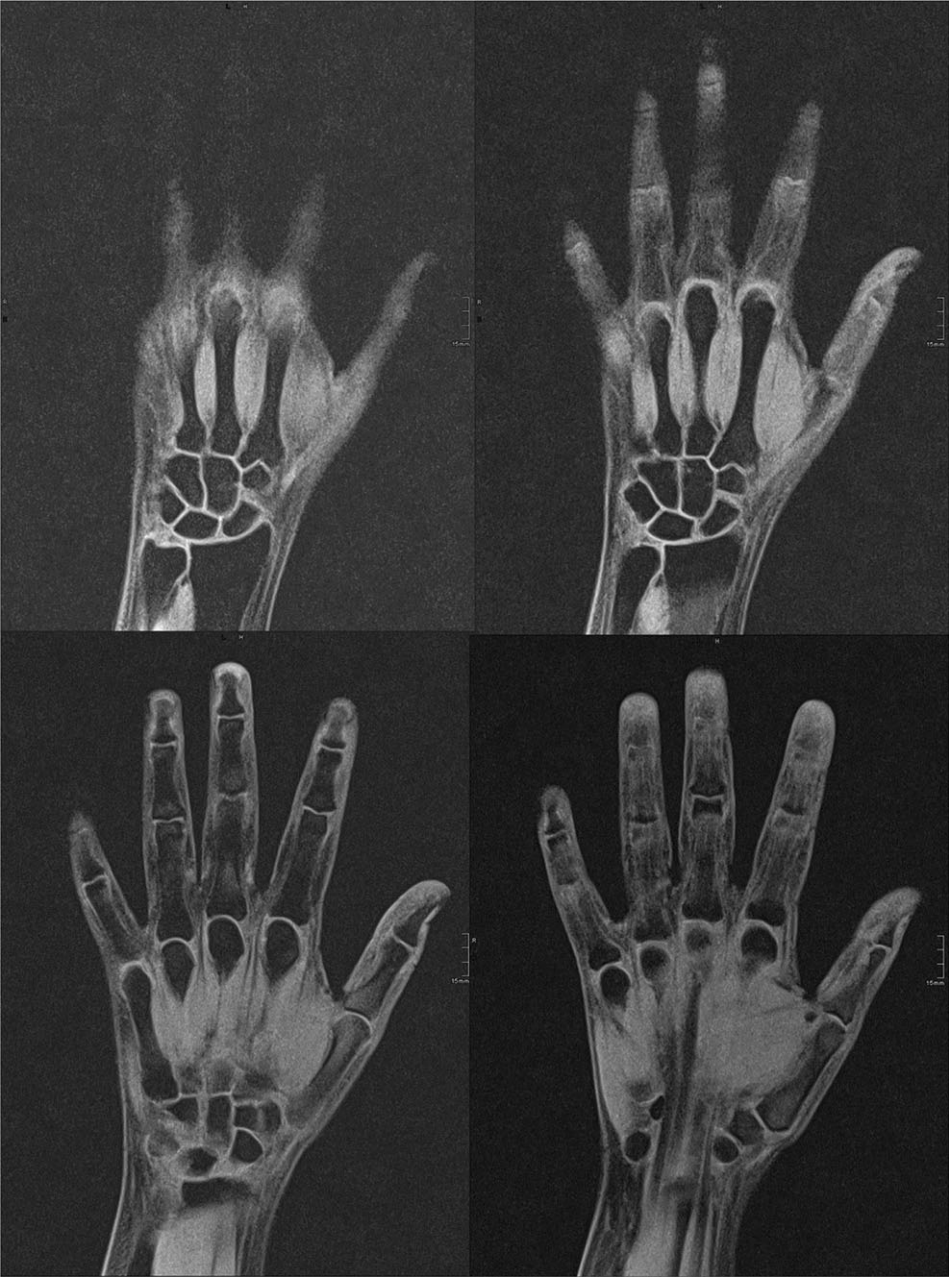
Four slices of a coronal T1 VIBE-3D-WE hand MRI of a 16-year-old healthy female. Estimated age was 18 years (reader 1) and 18 years (reader 2)
